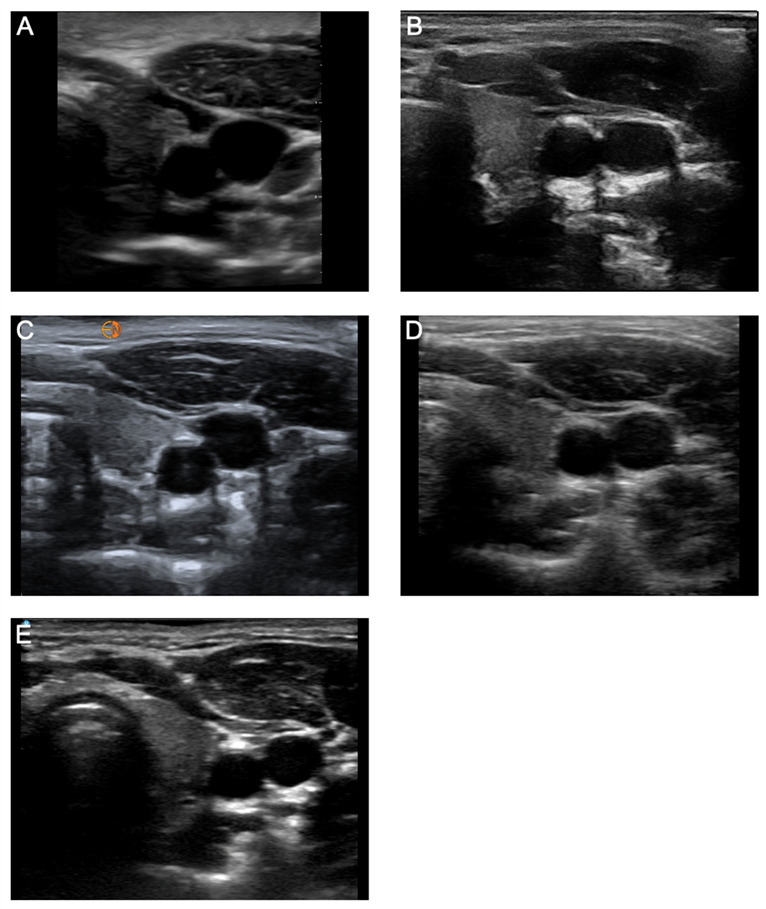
Lung Views of the same pediatric model using six handheld ultrasound devices: (A) Butterfly iQ+™, (B) Clarius®, (C) Kosmos™, (D) Lumify™, (E) Vscan Air™.
